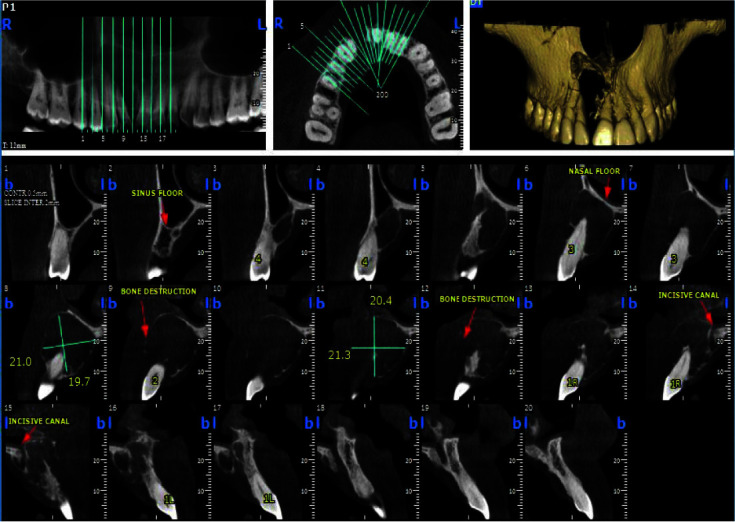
Cone beam computed tomography (CBCT) images show a well-defined corticated unilocular radiolucent lesion from maxillary right central incisor to the right first premolar
